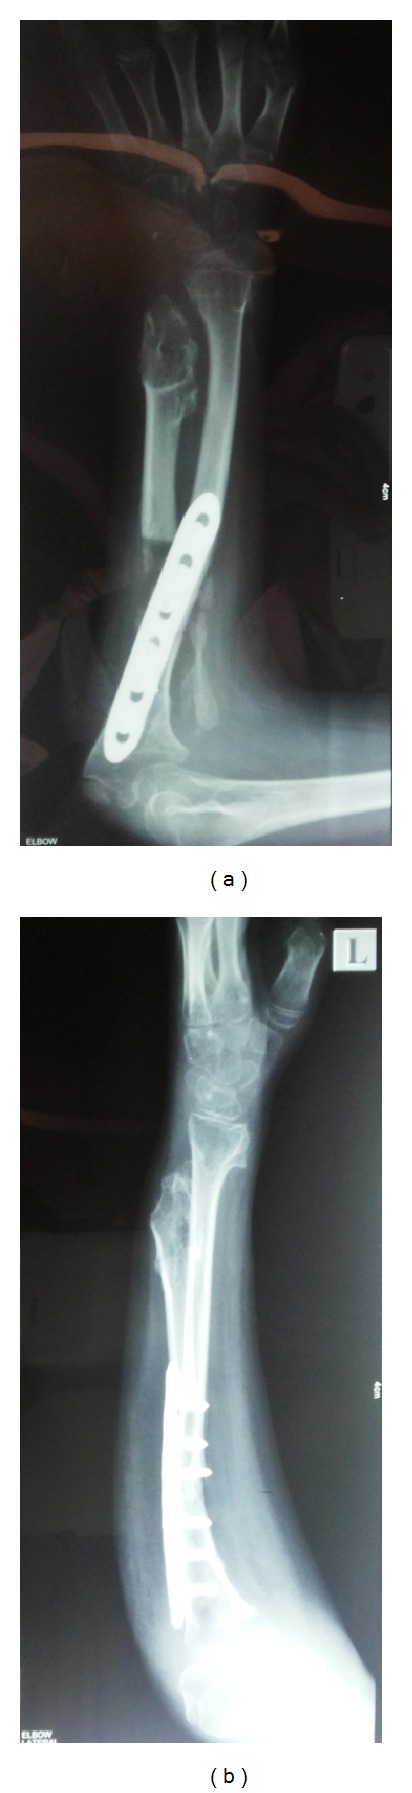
Followup at 3 months. X-ray showing union.
